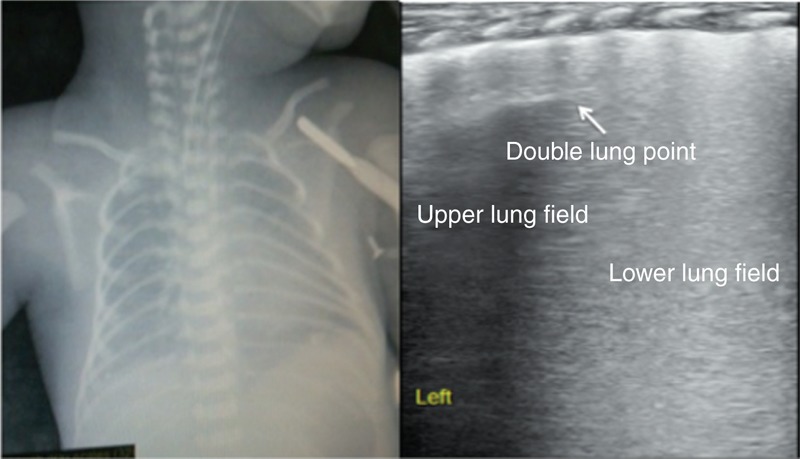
Ultrasonic manifestation of TTN: double-lung point. The infant above had a GA of 28^+1^ wk and was delivered vaginally; the infant had a birth weight of 1150 g. The infant was admitted to the hospital after suffering from dyspnea for 4 h. Lung ultrasonography showed clear double-lung point, interstitial lung syndrome, and fuzziness of the pleural lines. GA = gestational age, TTN = transient tachypnea of the newborn.
